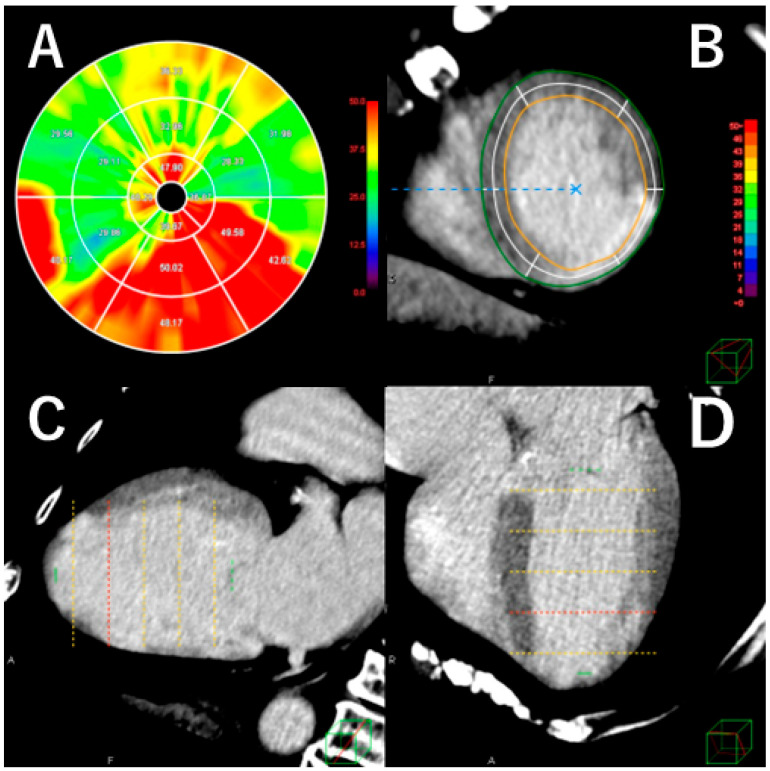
Left ventricular extracellular volume fraction (ECV) analysis on Computed tomography (CT). CT images in a 76-year-old female without reverse remodeling. Regional ECV elevation corresponds to a prior myocardial infarction related to atrial fibrillation–associated coronary embolism. ECV was calculated as the mean value across the 16 left ventricular segments (A). Quantitative ECV analysis was performed using short-axis, two-chamber, and four-chamber views (B–D). In (C,D), the colored dotted lines indicate the locations of short-axis slices used for ECV analysis, and the red dotted line corresponds to the slice displayed in (B).
